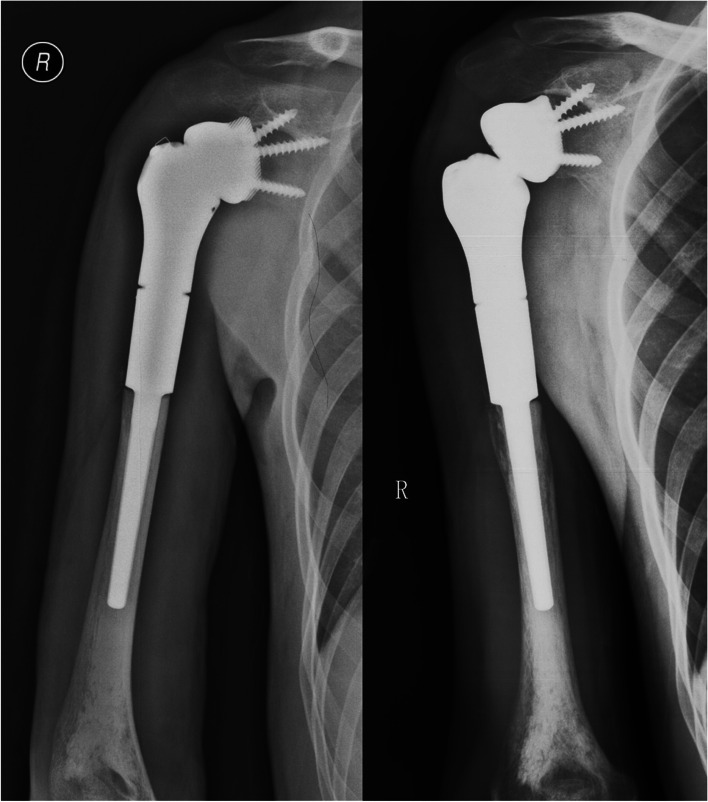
Detachment of the arthrodesis prosthesis for proximal humerus. A 20-year-old man diagnosed with osteosarcoma in the right proximal humerus underwent en bloc resection and prosthetic replacement. Postoperative X-ray showed good fixation of the prosthesis (left). Follow-up at 3 months showed detachment at the taper of the prosthesis, but the patient did not have any discomfort (right)
